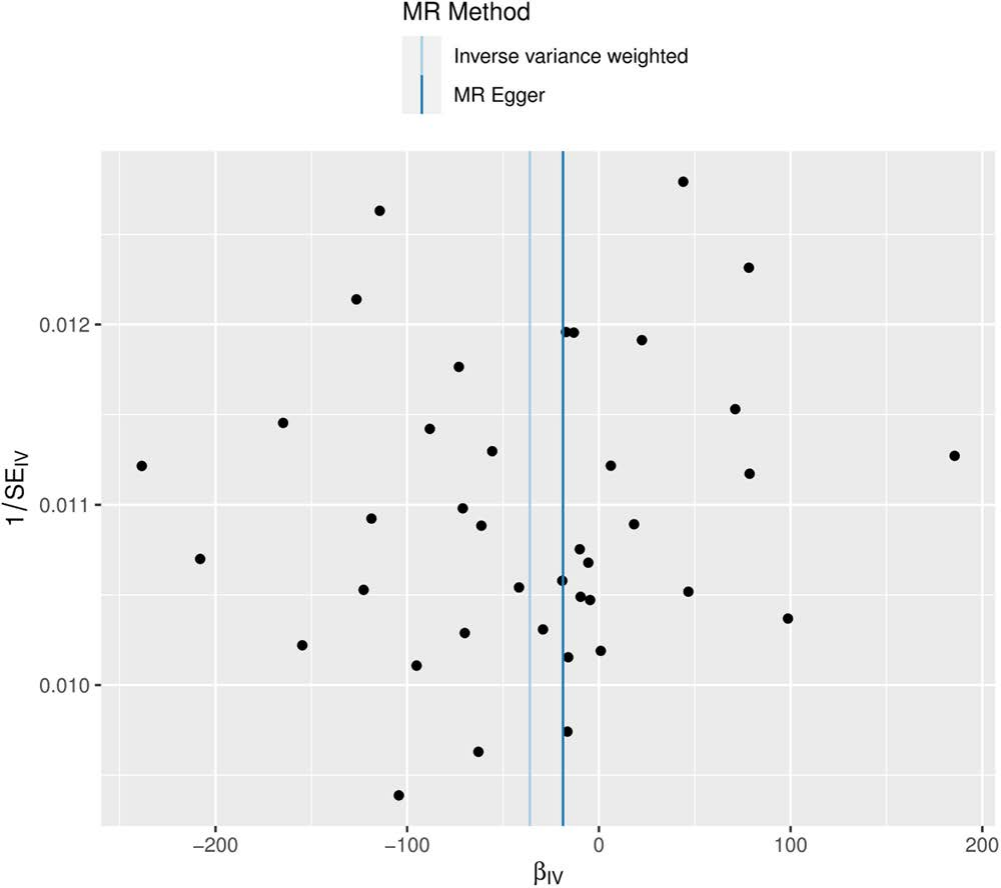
Funnel plot assessing potential directional pleiotropy. The symmetry of the funnel plot is used to visually evaluate the overall heterogeneity and potential directional pleiotropy among the instrumental variable estimates for the effect of CT scanning propensity on HCM risk. CT = computed tomography, HCM = hypertrophic cardiomyopathy, IVW = inverse-variance weighted.
